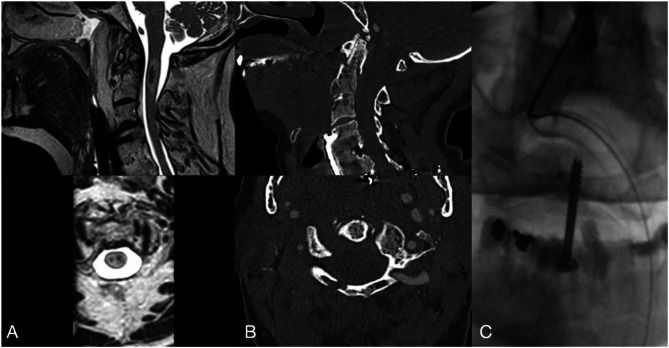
Case example patient number 2. 73 y/o hospitalized after a domestic fall from a staircase A top: sagittal and bottom: axial T2-weighted MRI imaging showing myelopathy (BASIC grade 4) without stenosis; B top: sagittal and bottom: axial CT imaging: showing posterior arch disruption of the atlas in an Anderson d’Alonzo type II (AO Type II B; M1-3) odontoid fracture with 3 mm displacement; C: intraoperative ap fluoroscopy of a ventral Dens axis screw; the patient regained consciousness on the ICU, was transferred to another ICU after two days, and survived
